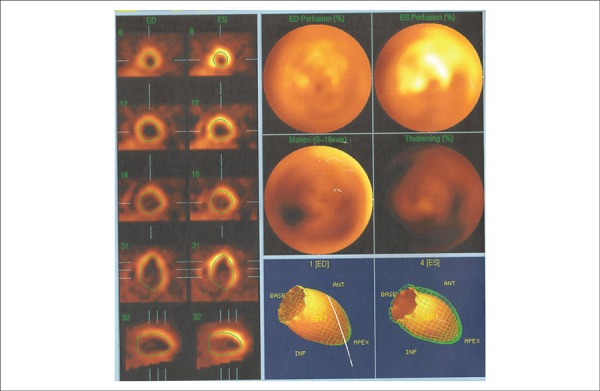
Nuclear ventriculography (gated SPECT scan): Diffuse left ventricular
